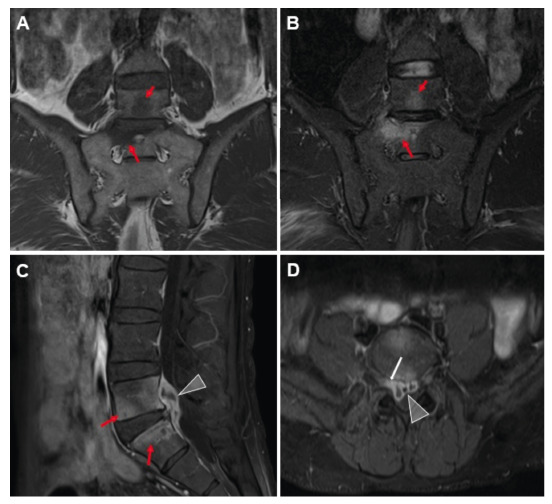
On magnetic resonance images, the L5 and S1 vertebral bodies appear hypointense on T1-weighted coronal images (A) and hyperintense on T2-weighted coronal images (B) (arrows). Post-contrast T1-weighted sagittal (C) and axial (D) images show increased contrast enhancement in the vertebrae (red arrows), a peripherally enhanced abscess focus in the epidural space (arrowheads), and right nerve root enhancement (white arrow).
